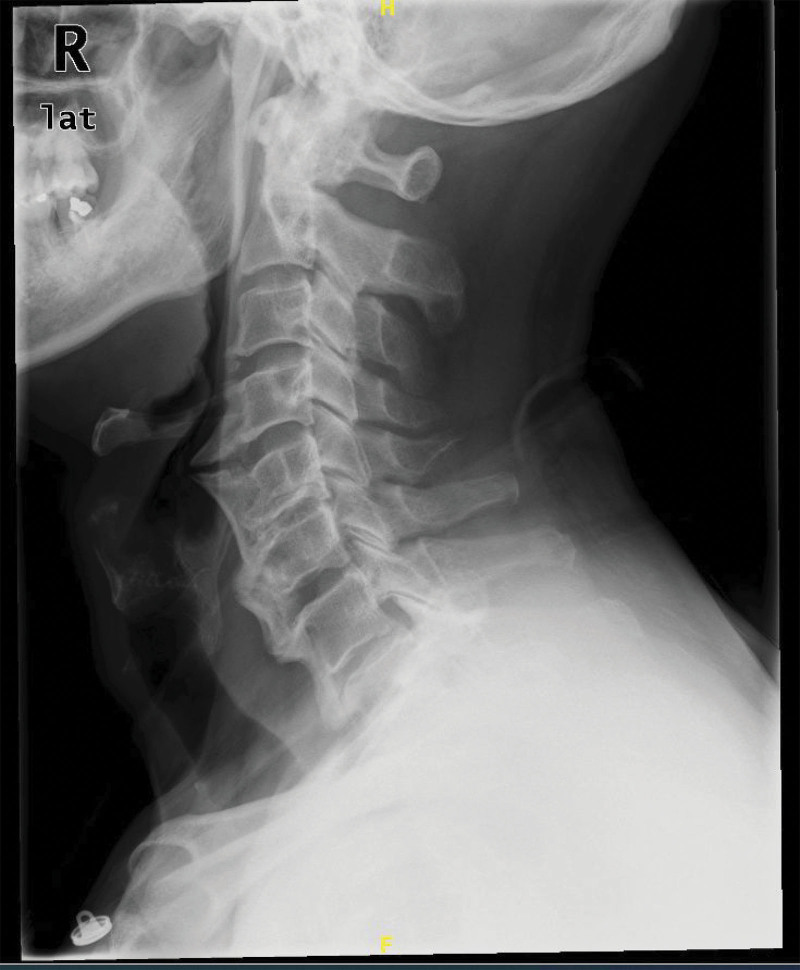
Preoperative lateral cervical X-ray showing significant calcification of the anterior longitudinal ligament and large osteophytes from C4 to C7 levels.
